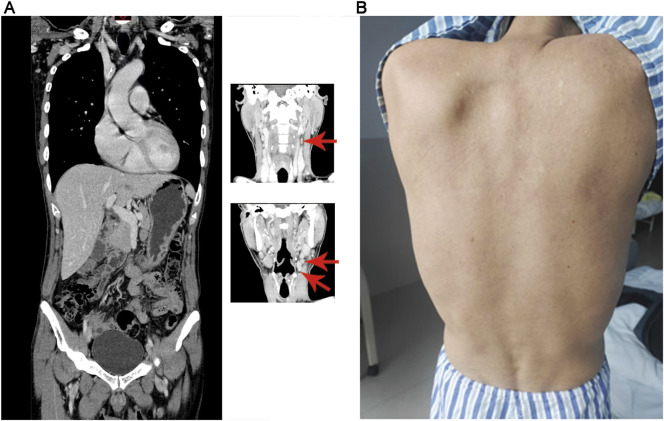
The imaging and cutaneous conditions after chidamide plus CHOP therapy. (A) CT demonstrating resolution of the previously noted lymphadenopathy. (B) Clinical appearance showing resolution of multiple subcutaneous nodules over the whole body.
